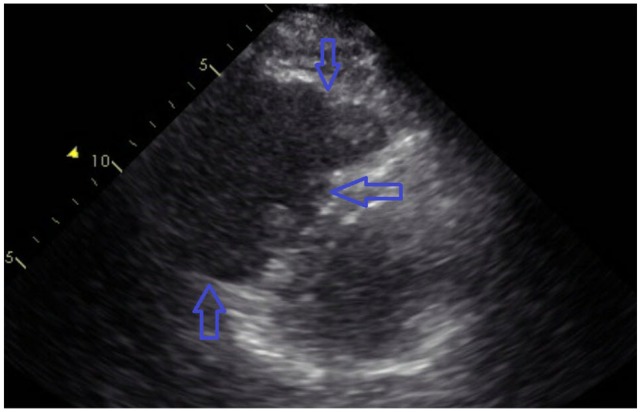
Echocardiogram showing right ventricular dilatation and hypokinesis.
